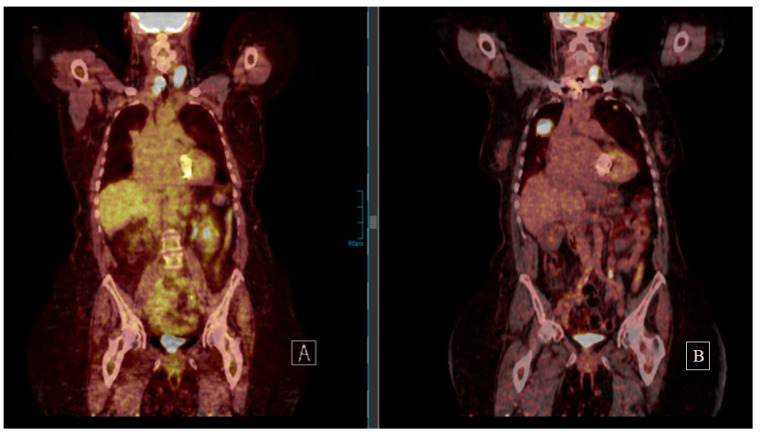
Patient 5 underwent resection of a cervical FTC recurrence diagnosed 5 years after thyroidectomy despite bilateral lung metastases (18F-FDG PET on the left side (A)). Cervical and thorax EBRT were performed. The 18F-FDG PET on the right (B) (5 years after the second cervical resection and 10 years after first FTC diagnosis) shows both cervical and lung progress. After thorough patient information concerning the evidence on tyrosine kinase inhibitors, the patient refused treatment and was progressing at last follow-up.
